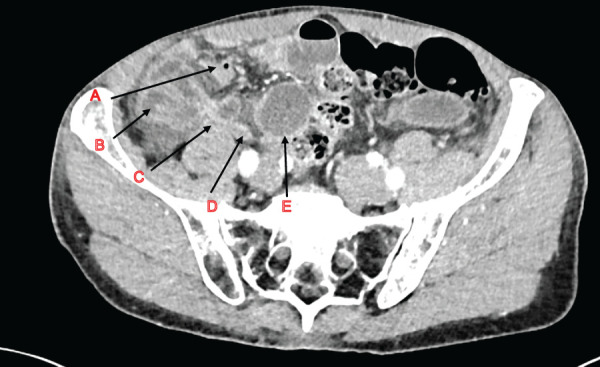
Preoperative CT scan showing (arrows): (A) gas-filled appendix, (B) incarcerated bowel loop, (C) hernial orifice, (D) flattened distal bowel loop, and (E) dilated proximal bowel loop.
